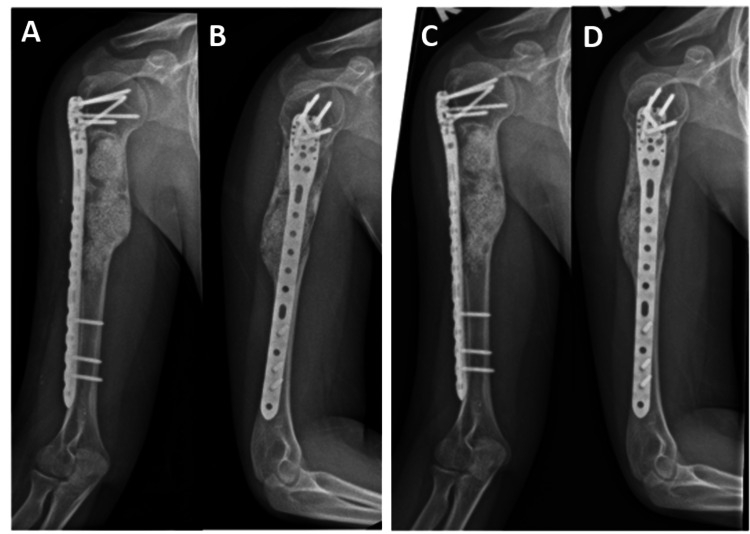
Radiograph of right humerus A: Anteroposterior view three months postoperatively, B: Lateral view three months postoperatively, C: Anteroposterior view six months postoperatively, D: Lateral view six months postoperatively
